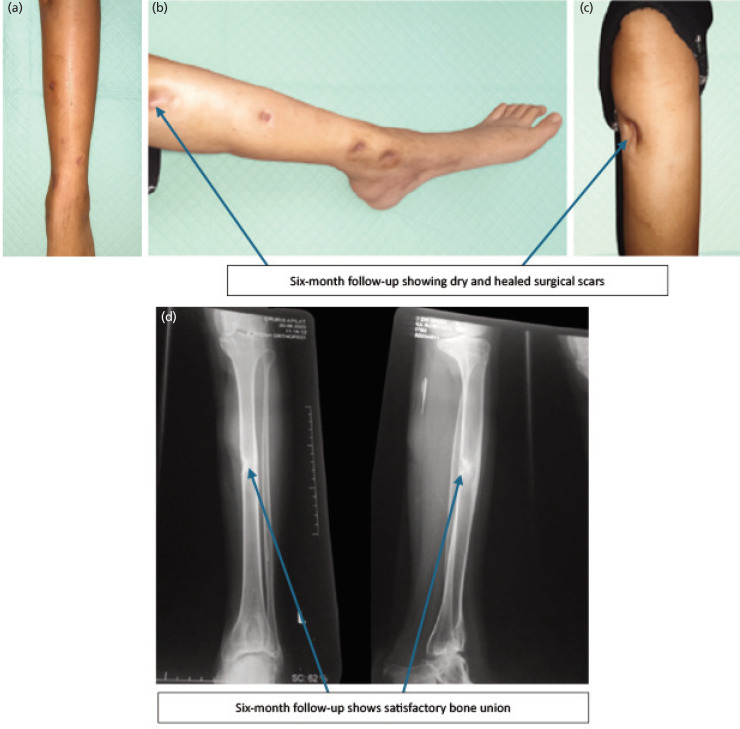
(a) Clinical and radiological appearance at six-month follow-up. Anterior view of the left lower leg showing well-healed surgical scars. (b and c) Medial and lateral views showing no signs of active infection and complete wound closure. (d) Anteroposterior and lateral radiographs demonstrating satisfactory bone union.
